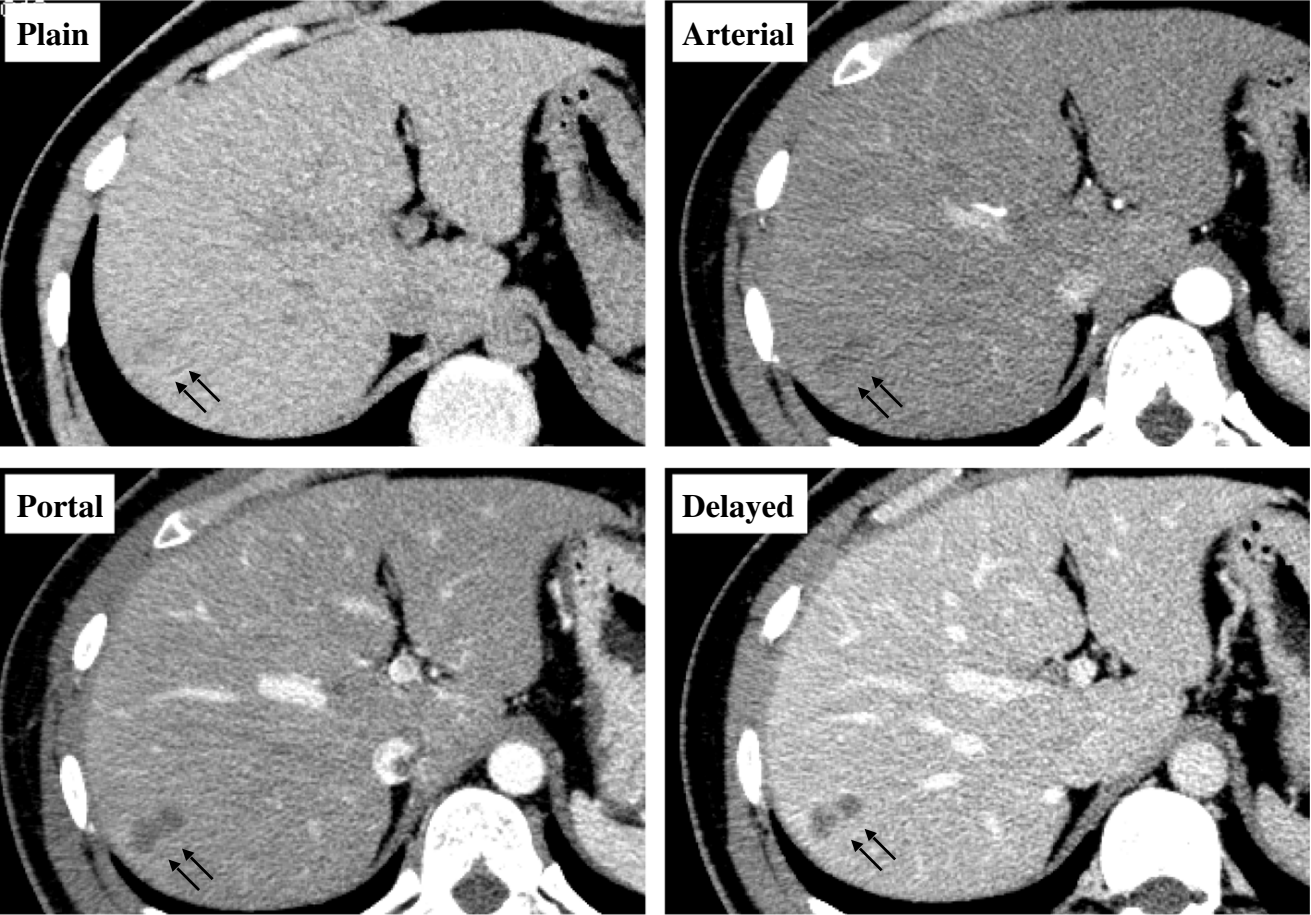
Axial slice on dynamic enhanced CT before operation. A solitary hypovascular mass detected in segment 7 of the liver (black arrows)
